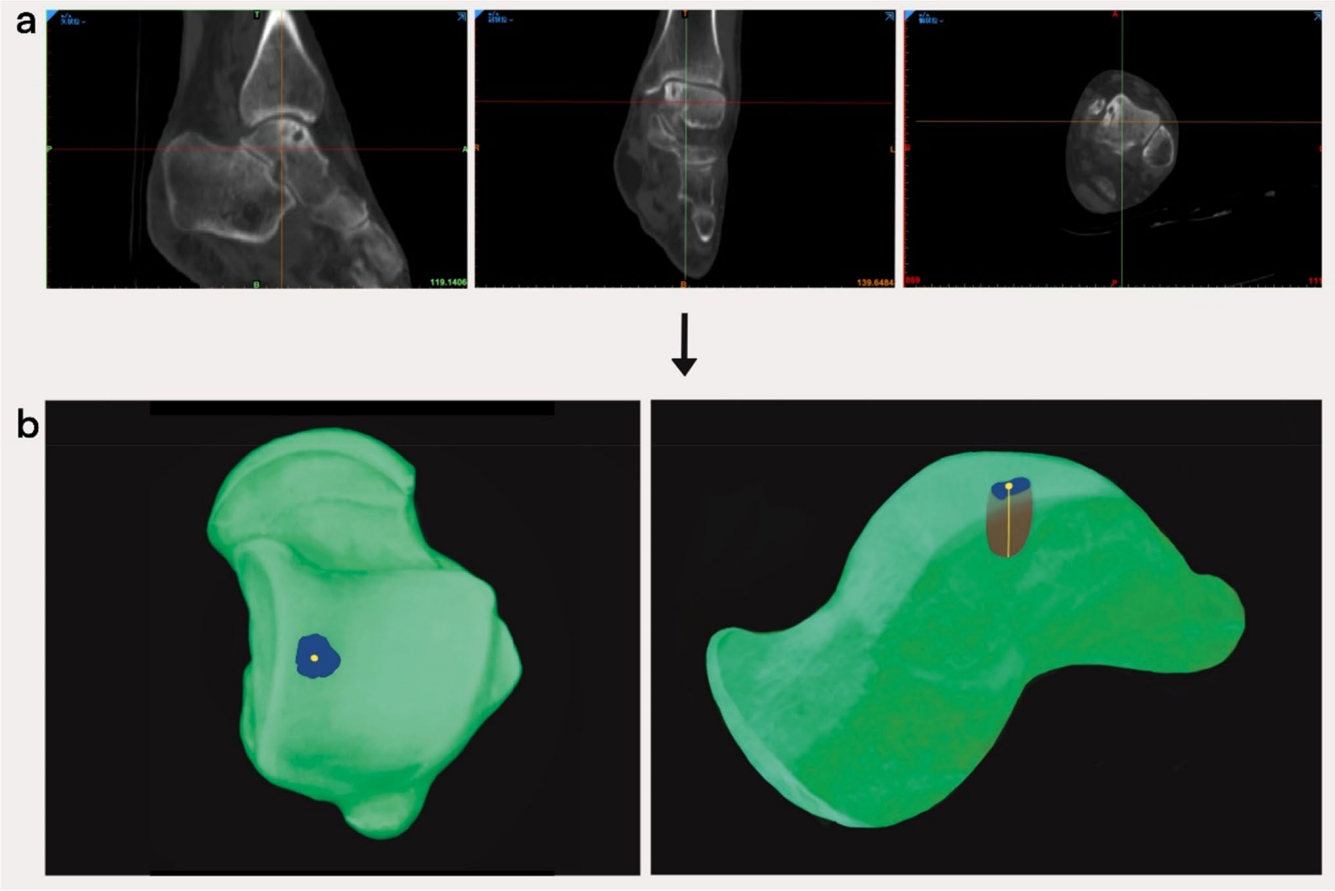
Annotation and measurement of the lesion site. a The location of the talus osteochondral injury was determined by CT sagittal/coronal/axial views. b The injury surface area (blue area) was measured in the three-dimensional view, a vertical line was drawn from the center of the injury surface area to the bottom of the cyst (yellow area) to accurately measure the depth of the injury, and the cystic area was perfused. The red area in the figure is the cystic volume
